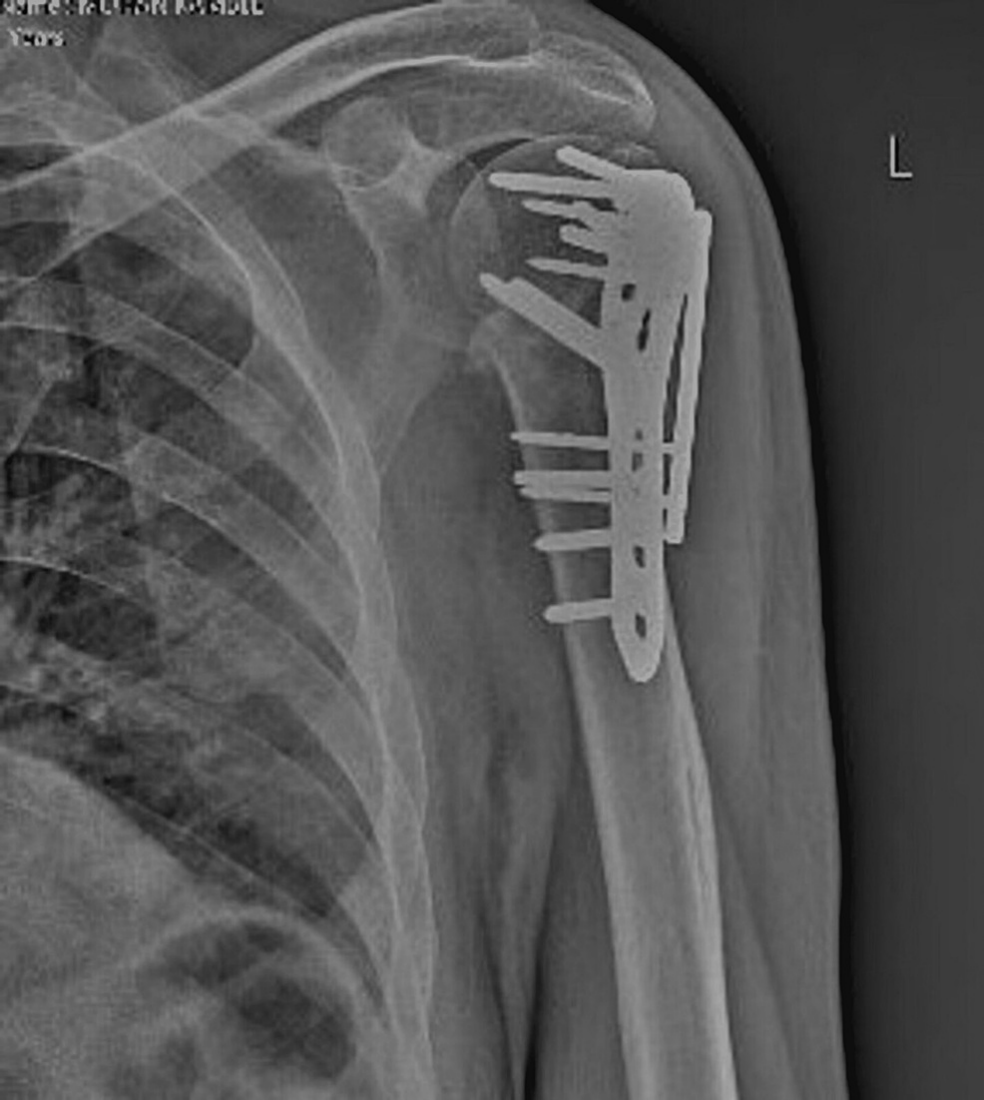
Follow-up plain radiograph antero-posterior view at three-month post-operatively
